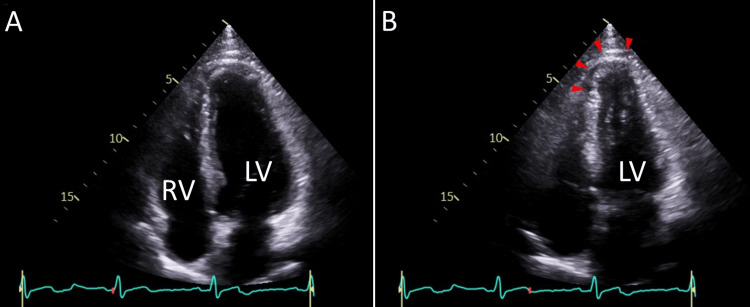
Echocardiography Echocardiographic apical four-chamber views at end-diastole (A) and end-systole (B) show mildly reduced wall motion in the anteroseptal wall and apex of the left ventricle (LV) (arrowheads). RV denotes the right ventricle.
